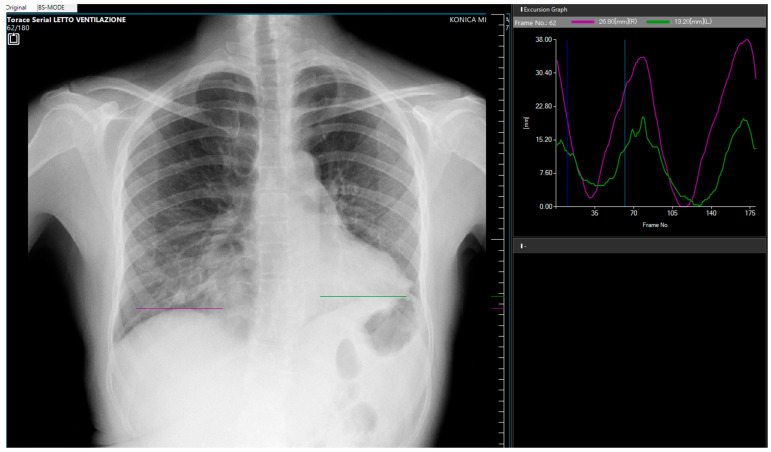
Posteroanterior Dynamic Digital Radiography (DDR) during forced breathing (AeroDR TX, Konica Minolta Inc., Tokyo, Japan). Acquisition parameters were: tube voltage, 100 kV; tube current, 50 mA; 1.6 ms; source-to-image distance, 2 m; additional filter, 0.5 mm Al +0.1 mm Cu; exposure, 12 s; 15 frames/s. The pixel size was 388 × 388 μm, the matrix size, 1024 × 768, and the overall image area 40 × 30 cm. Diaphragmatic motion was assessed using the dedicated Konica Minolta workstation software (DX-1), which allows for a dynamic review of sequential images in cine mode. The purple curve represents the right diaphragm’s motion, and the green curve represents the left diaphragm’s motion [1]. Note the reduced excursion of the green curve, indicating impaired left diaphragm motility (Supplementary Materials S1, S2). The vertical displacement of the left hemidiaphragm was visually assessed relative to the contralateral side during deep breathing. While quantitative motion analysis software is available, in this case, the assessment was based on qualitative grading of diaphragm excursion reduction compared to the contralateral (right) hemidiaphragm within the same acquisition. Diaphragm dysfunction diagnosis is quite challenging, mainly based on medical history (symptoms such as shortness of breath, especially when lying down, unexplained fatigue, or reduced exercise tolerance) and physical exam (decreased breath sounds at the lung bases) [2]. A standard X-ray can reveal an elevated hemidiaphragm, which may suggest paralysis or dysfunction. Ultrasound may allow direct visualization of the diaphragm and its motion but is strictly related to the operator’s experience. CT or MRI provide detailed anatomy but no dynamic functional assessment [3,4]. Unlike traditional static imaging, DDR provides dynamic visualization of diaphragm movement during respiration, allowing for a more accurate assessment of diaphragm motility and function [1,4]. This technique captures a series of high temporal resolution images during patient breaths, generating a motion study of the diaphragm [1]. This allows for visualization of the diaphragm’s excursion, speed, and symmetry during inspiration and expiration [2]. The use of pulsed X-ray allows a lower radiation exposure when compared to fluoroscopy [1]. The post-processing also provides information on the motility in different areas of the lungs [5]. The right lung shows normal motility, with a well-represented motion in the lower field. The motility of the left lung is instead reduce, with fewer and smaller green arrows (as shown in Supplementary Materials S2).
